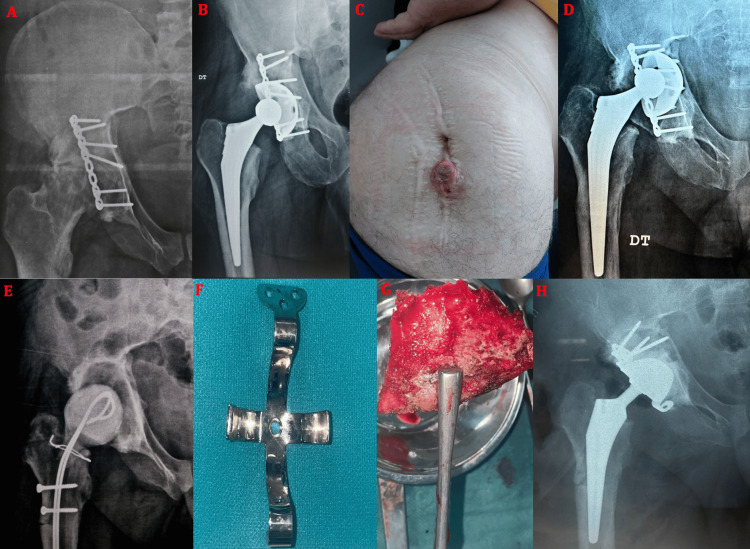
Case of a 46-year-old male patient from the series. (A) Anteroposterior radiograph of the right hip showing post-traumatic hip osteoarthritis associated with avascular necrosis of the femoral head following an acetabular fracture initially treated with plate-and-screw fixation. (B) The patient subsequently underwent cementless double-mobility total hip arthroplasty. Clinical evolution was marked by the development of a draining sinus with purulent discharge 14 months after THA implantation (C), associated with aseptic loosening of the acetabular cup (D). The patient was initially treated with surgical debridement, removal of the prosthesis, placement of an antibiotic-loaded spacer, and targeted antibiotic therapy, followed by secondary reconstruction using a Kerboul acetabular reinforcement ring (F) combined with corticocancellous autograft (G), as demonstrated on the final follow-up radiograph (H).
